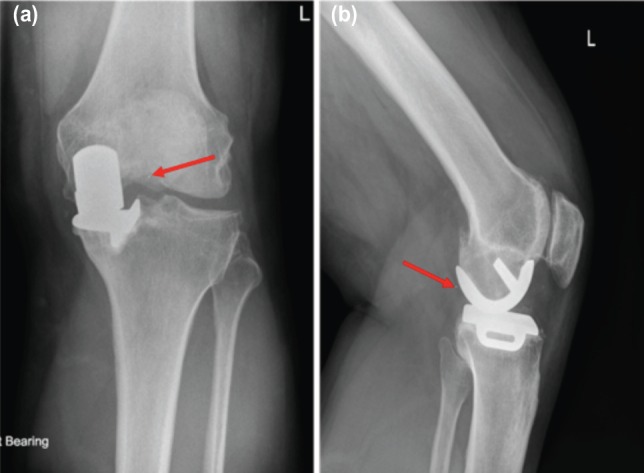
(a) AP and (b) Lateral radiographs with arrows showing dislocated meniscal bearing insert.
